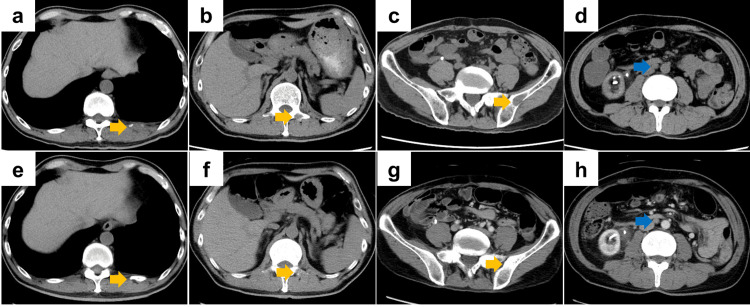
Computed tomography (CT) findings in Case 1 (a-d) Baseline contrast-enhanced CT at the time of diagnosis of metastatic disease. (a) Lytic lesion in the right 11th rib, (b) osteolytic lesion in the second lumbar vertebra, (c) osteolytic lesion in the left iliac bone, and (d) para-aortic lymph node metastasis. (e-h) CT after 4.2 months of first-line enfortumab vedotin plus pembrolizumab. The metastatic lesions in the 11th rib and left iliac bone are reduced in size, whereas the second lumbar vertebral lesion and para-aortic lymph node metastasis remain radiologically stable. Yellow arrows indicate bone metastases. The blue arrow indicates a para-aortic lymph node metastasis.
